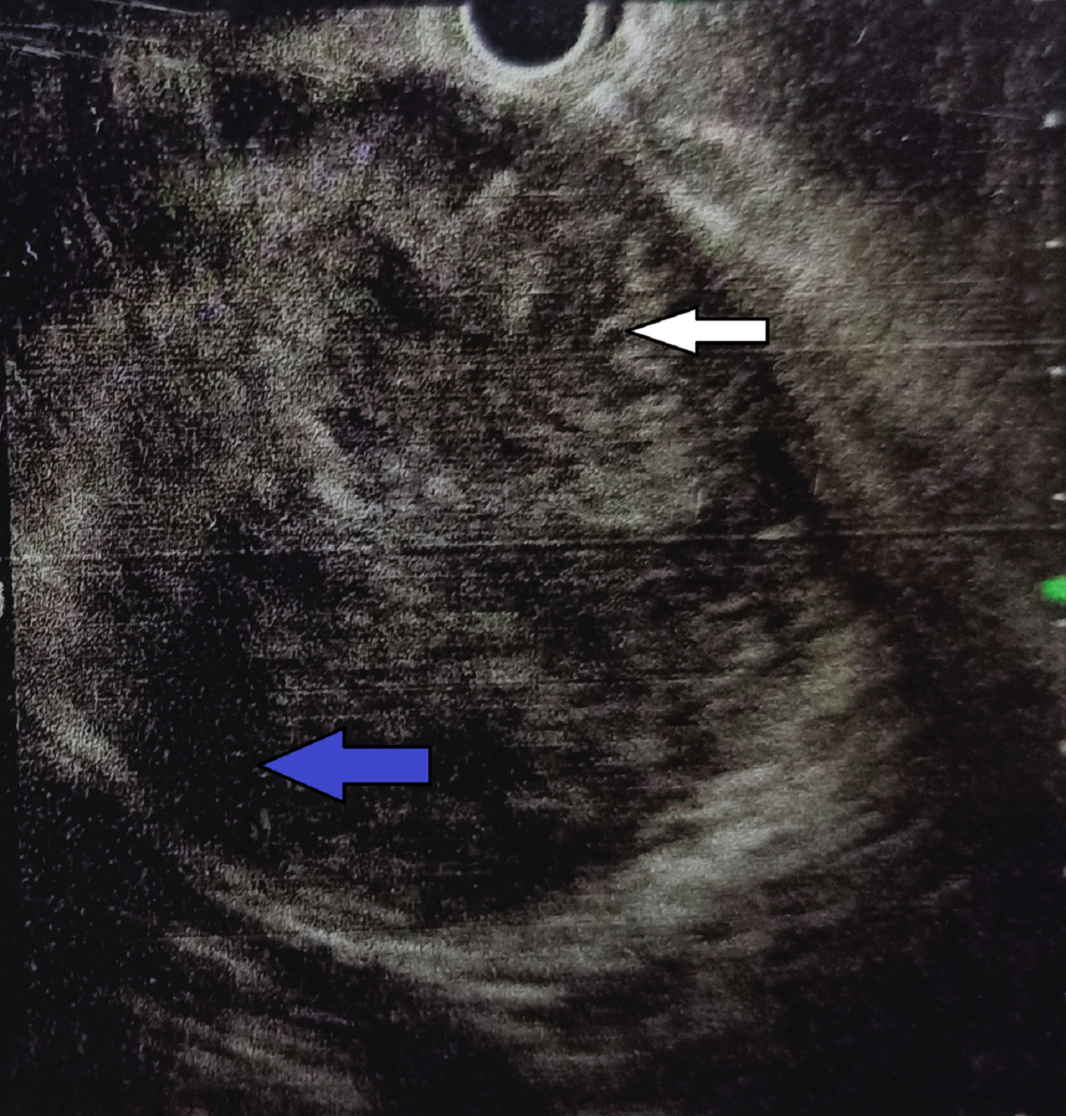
EUS picture showing solid component (marked with white arrow) with cystic degeneration (marked with blue arrow) EUS: endoscopic ultrasound
